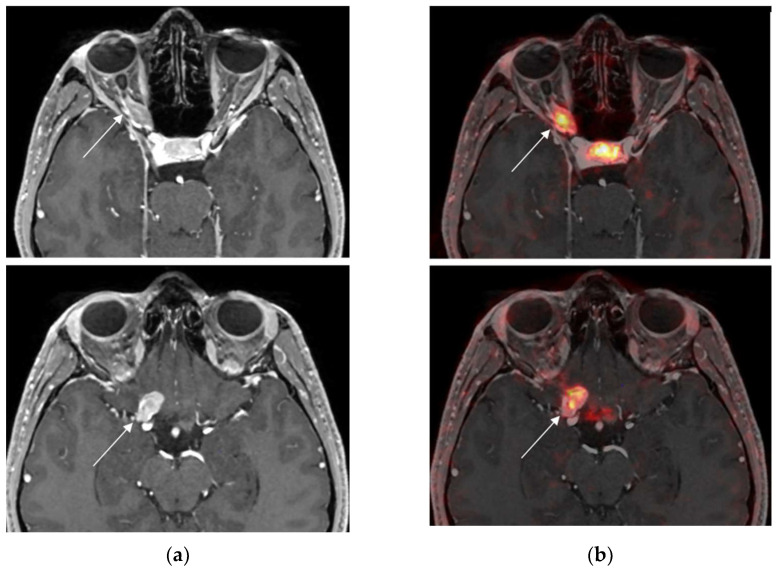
Case 2. Axial MRI T1WI (a) showing enlargement with enhancement of the right intraorbital (top row) and prechiasmatic tumours (bottom row) (white arrow). Axial fused MRI and ^68^Ga-DOTATOC PET images (b) showing intense tracer uptake in the right intraorbital tumour (white arrow): tumour SUV_max_ = 6.9; pituitary SUV_max_ = 9.4.
